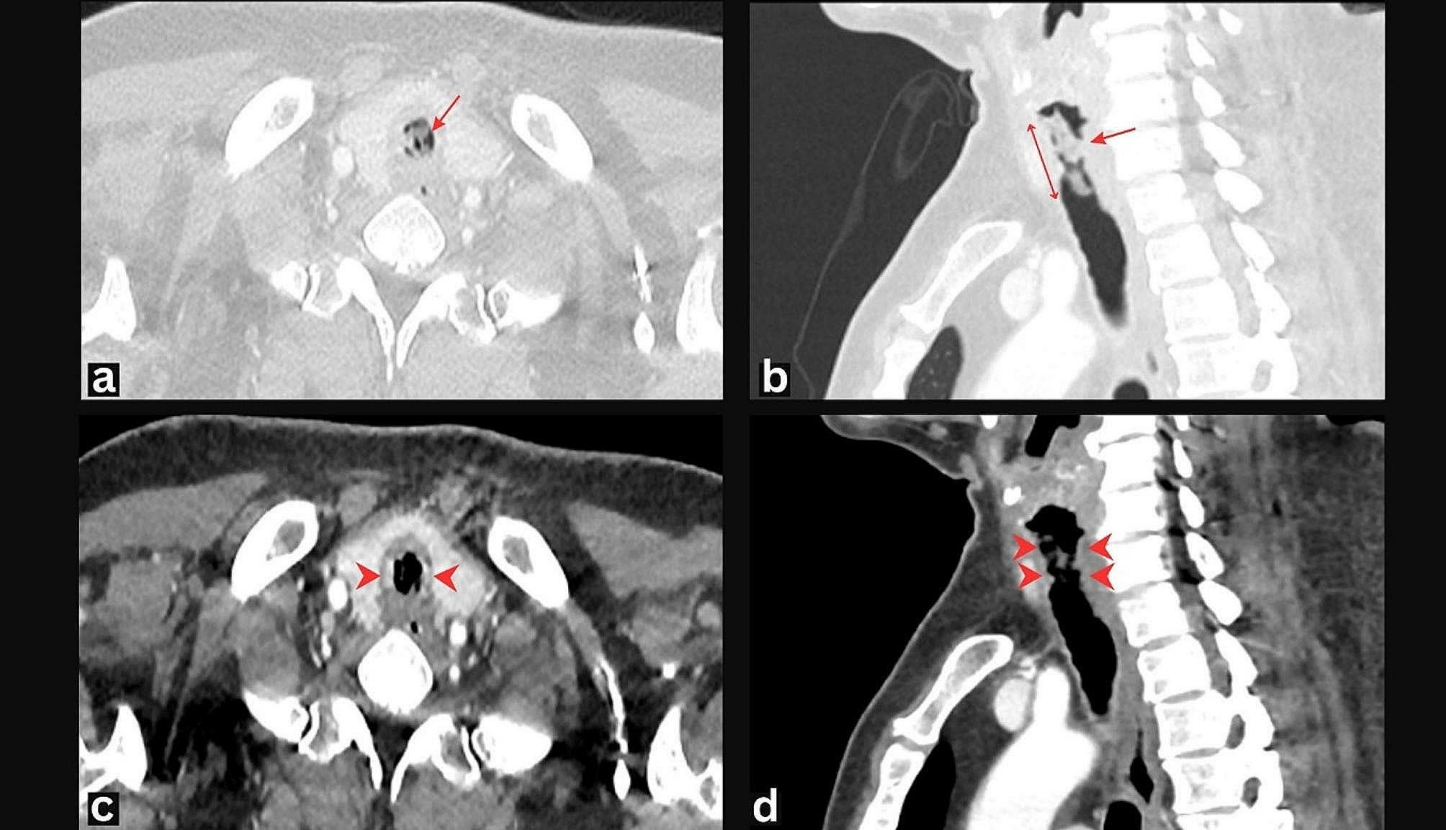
(a-d) Contrast enhanced CT of the neck and thorax in axial and sagittal view at thyroid level. Lung window (a and b) demonstrates a focal, short-segment endoluminal mucous secretion within the trachea (arrow) at the subglottic region, along a length of 3.5 cm (double arrow). The tracheal wall is irregular with circumferential thickening (arrowhead) and luminal narrowing on soft tissue window (c and d)
